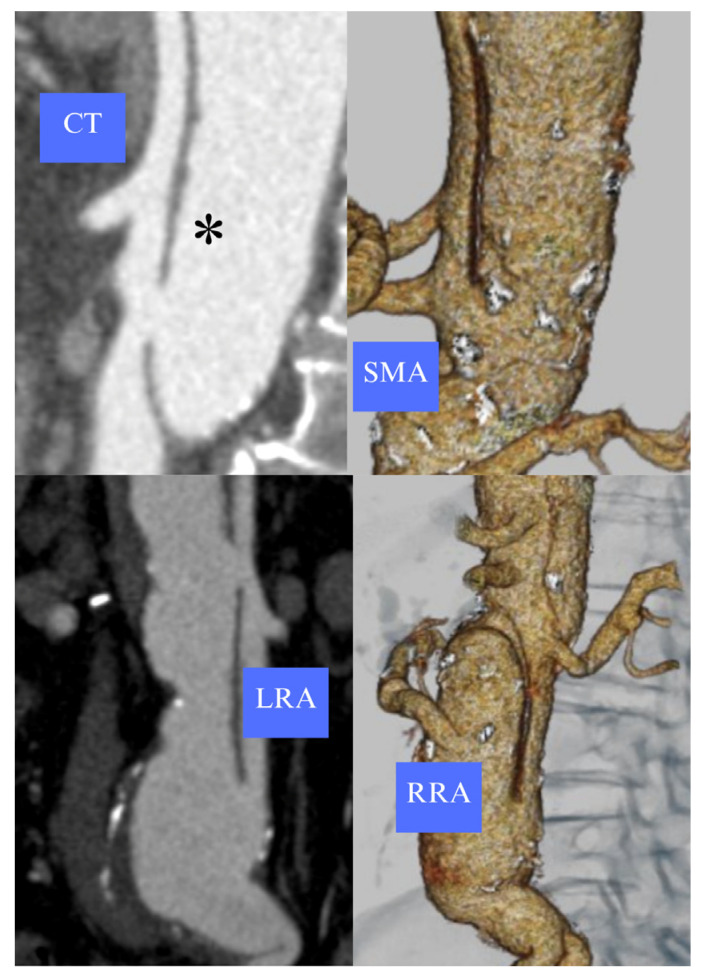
Anatomical features of visceral vessels of a post-dissection type IV TAAA. The dissecting lamella (*) separates the true and false lumen. MPR and 3D reconstructions show an upward-oriented RRA originating from a dilated false lumen, while the CT, SMA, and LRA arise from a narrow true lumen.
